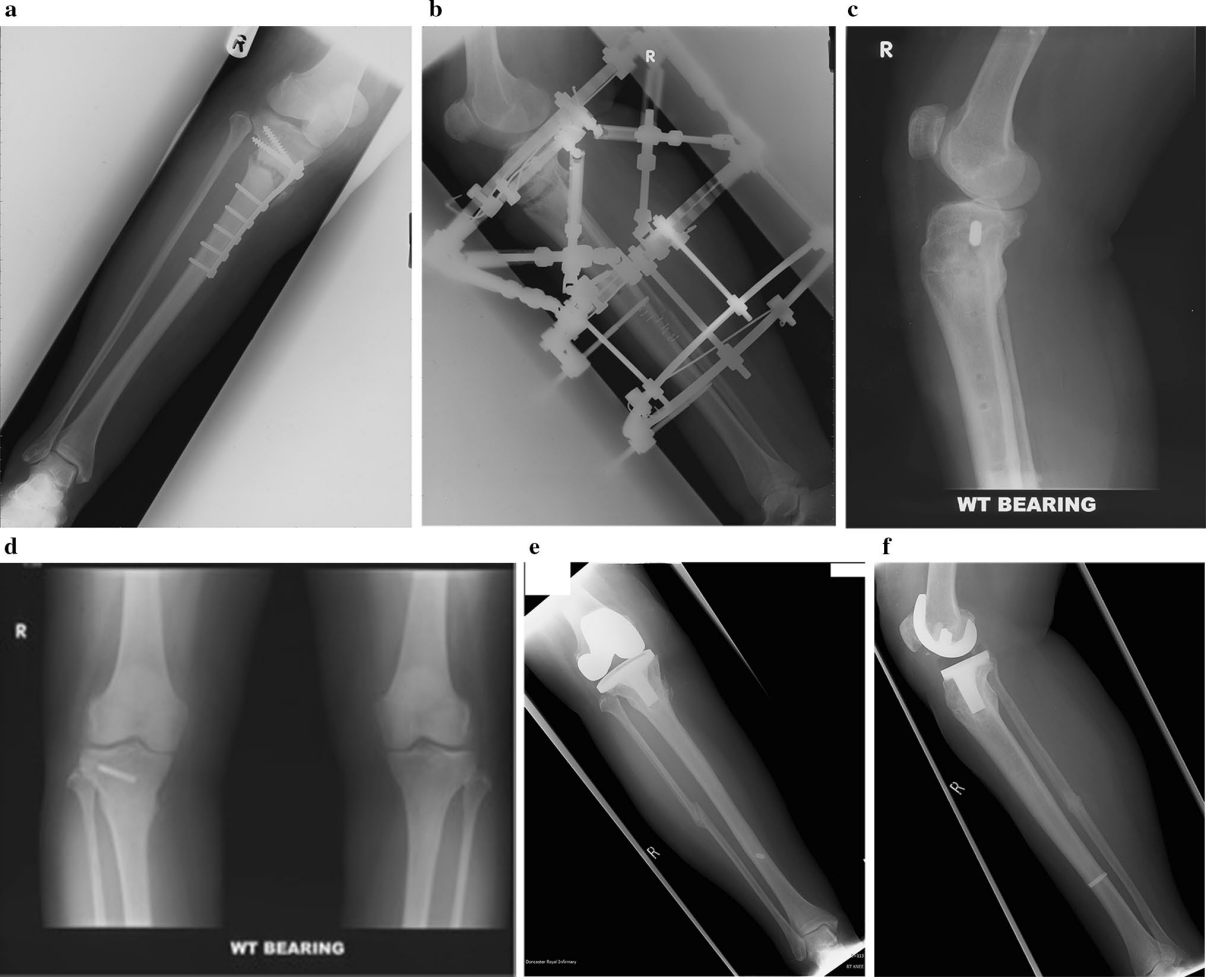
Case 1: a Radiograph showing established proximal tibial non-union following stress fracture, failed treatment of internal fixation and bone graft augmentation. b Radiograph showing frame fixation for treatment of proximal tibial non-union and deformity correction. c, d Orthogonal radiographs showing union of the stress fracture. e, f Orthogonal radiographs showing total knee replacement in situ performed as a staged procedure
